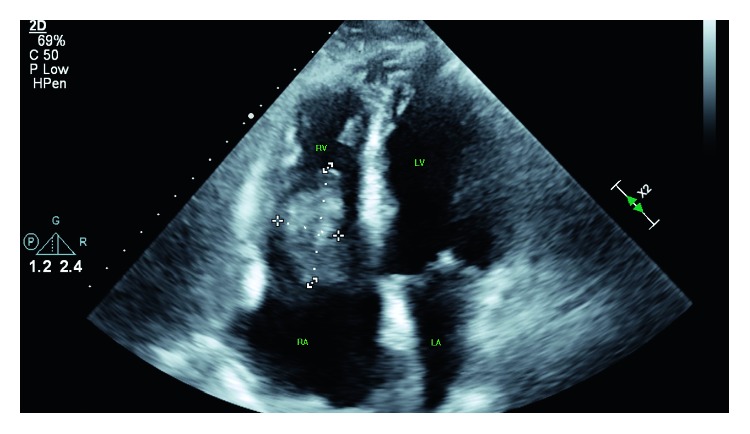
Transthoracic echocardiogram showing a mass involving the tricuspid valve and both the right atrium and ventricle.
